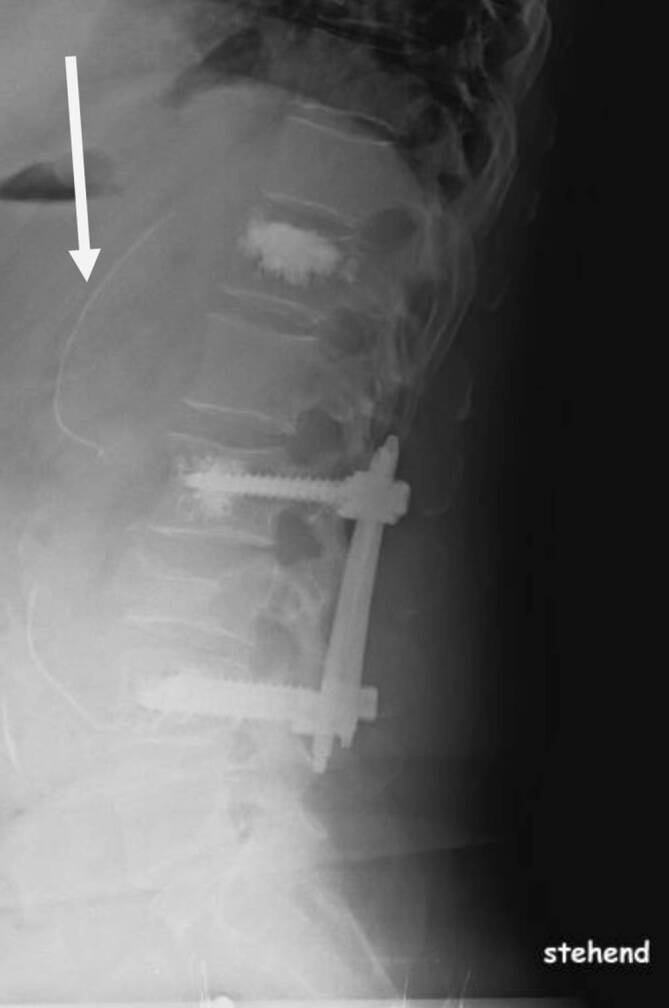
Das postoperative CT der LWS bestätigte die vermutete Zementembolie. Diese ging von den rechtsseitigen Pedikelschrauben in LWK 2 und 4 aus und erfolgte über paravertebrale Venen in die V. cava inferior. Die Schraubenlage und Frakturreposition waren regelrecht. Die Zementfahnen hatten Verbindung zu den Wirbelkörpern (Abb. 3). Eine klinische Symptomatik bezüglich einer Zementlungenembolie bestand nicht. Es erfolgte eine Thromboseprophylaxe mit Dalteparin 5000 I.E. einmal täglich.
